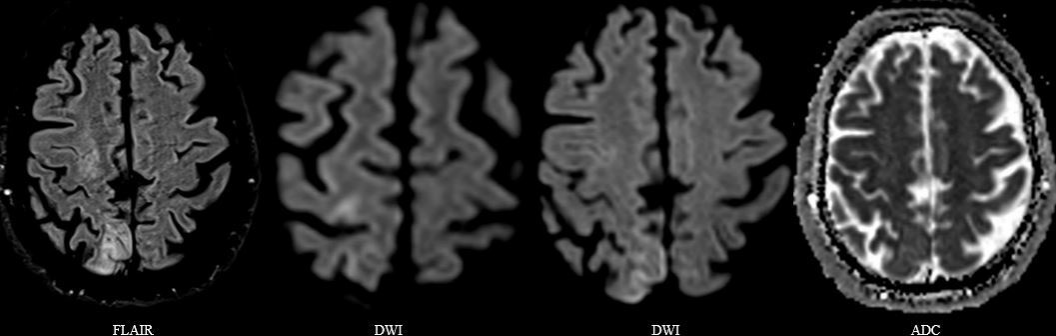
Cerebral MRI, with pathological T2-signals in the frontoparietal region and increased signal intensity on Diffusion-Weighted Images (DWI) and Apparent Diffusion Coefficient (ADC) representing vasogenic oedema. There is no visible infarcted tissue.
